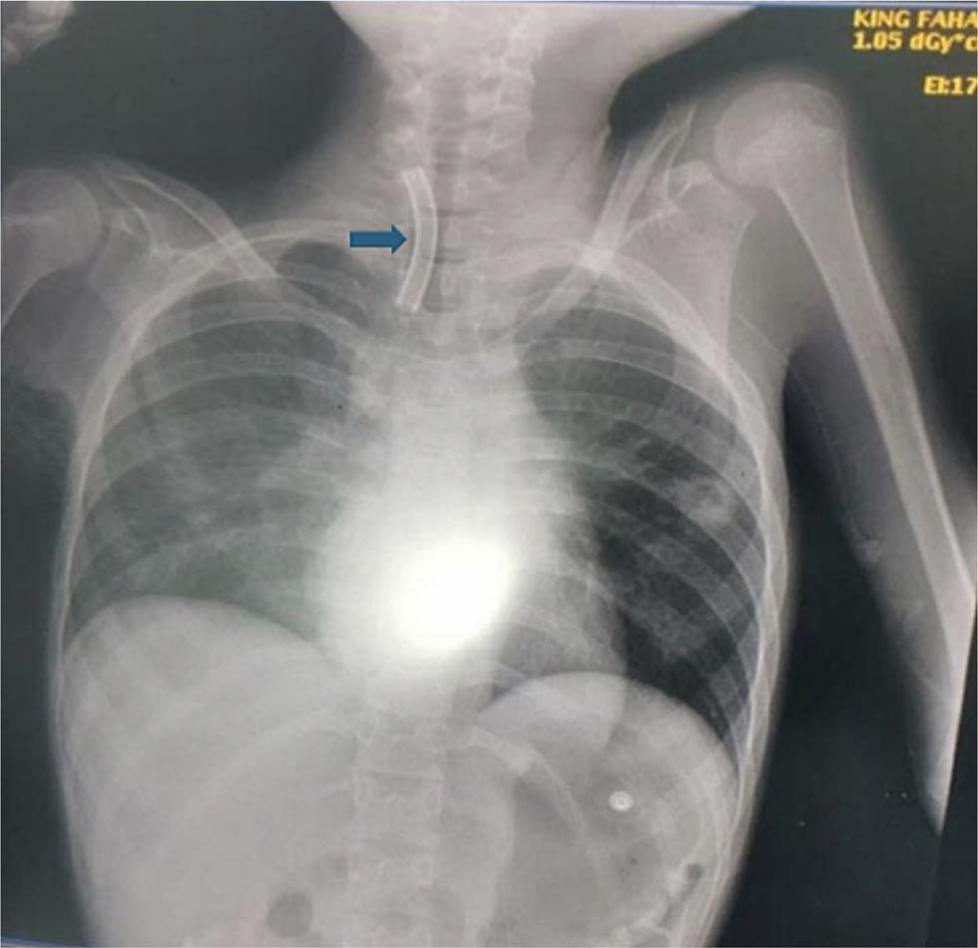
This figure demonstrates the patient chest X-ray showing the radio-opaque cannula in the right main bronchus (arrow).
